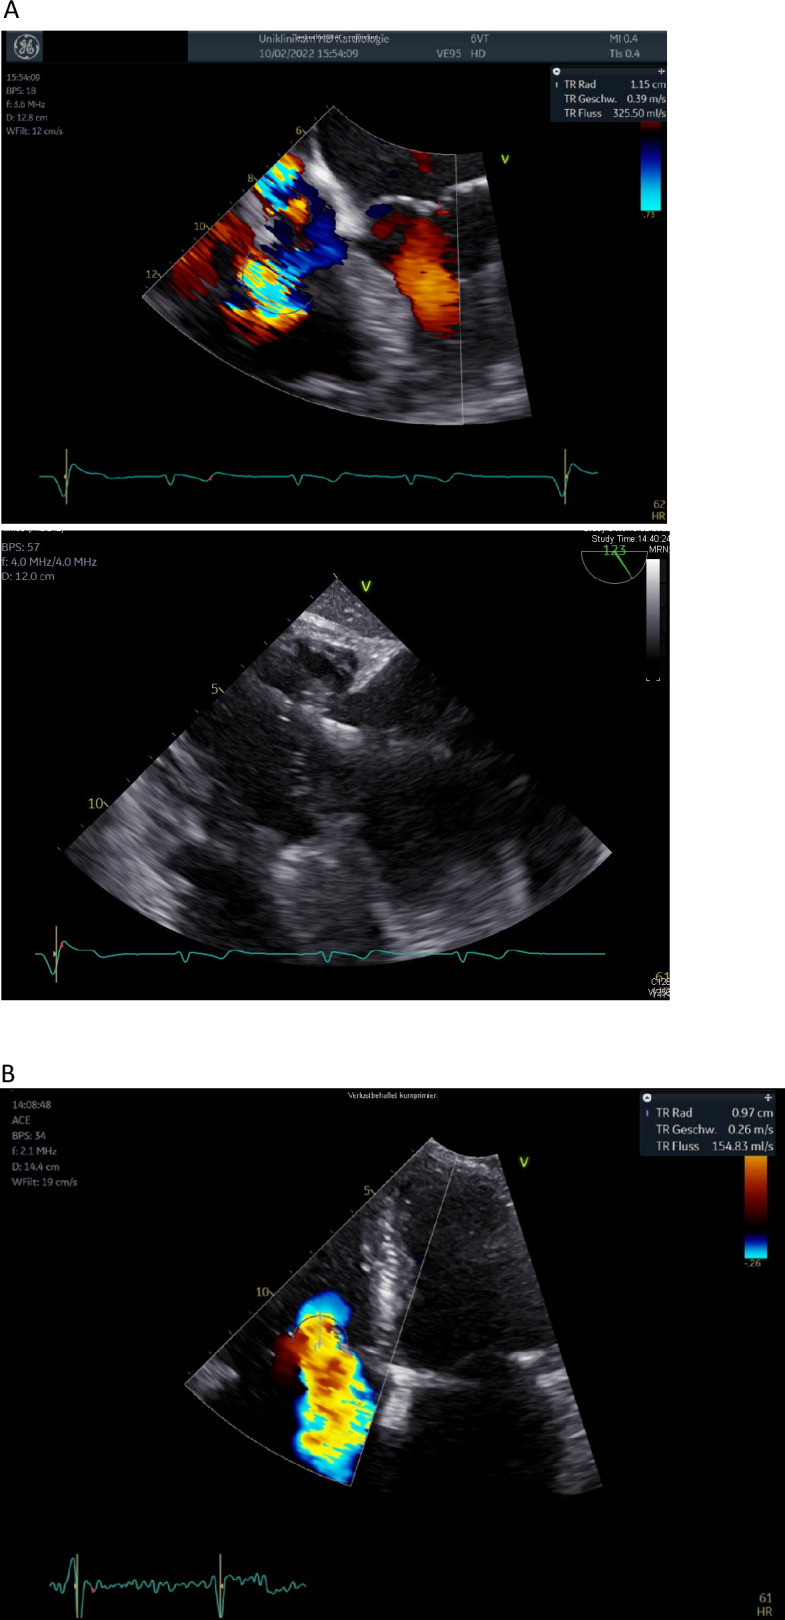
A Transoesophageal echocardiography before lead extraction. Shown here is the mechanical contribution of the electrode to the regurgitation by hindering leaflet closure as well as the Doppler signal of the regurgitation (PISA radius 1.53). B Echocardiography after lead extraction demonstrating a significant reduction of tricuspid regurgitation after lead extraction (PISA radius 0.97)
